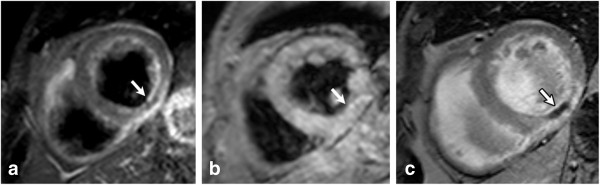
Shown are short axis images from a 48-year-old gentleman who presented with inferior ST-elevation and received primary percutaneous intervention to the RCA. CMR on day 2 post MI demonstrated an area of hypointense signal on T2-weighted imaging (Panel a), but no signal hypointensity on T2* imaging (Panel b). This area was confirmed to represent MO on early gadolinium imaging (Panel c). Intramyocardial hemorrhage results in decreased signal intensity on T2* imaging, and the absence of this feature reflects a lack of visible hemorrhage within the area of MO. (Image provided courtesy of Ananth Kidambi, BMBCh; Adam N. Mather, MBBS; and Sven Plein, MD, PhD; Multidisciplinary Cardiovascular Research Centre & Leeds Institute of Genetics, Health, and Therapeutics, University of Leeds, Leeds, United Kingdom.).
